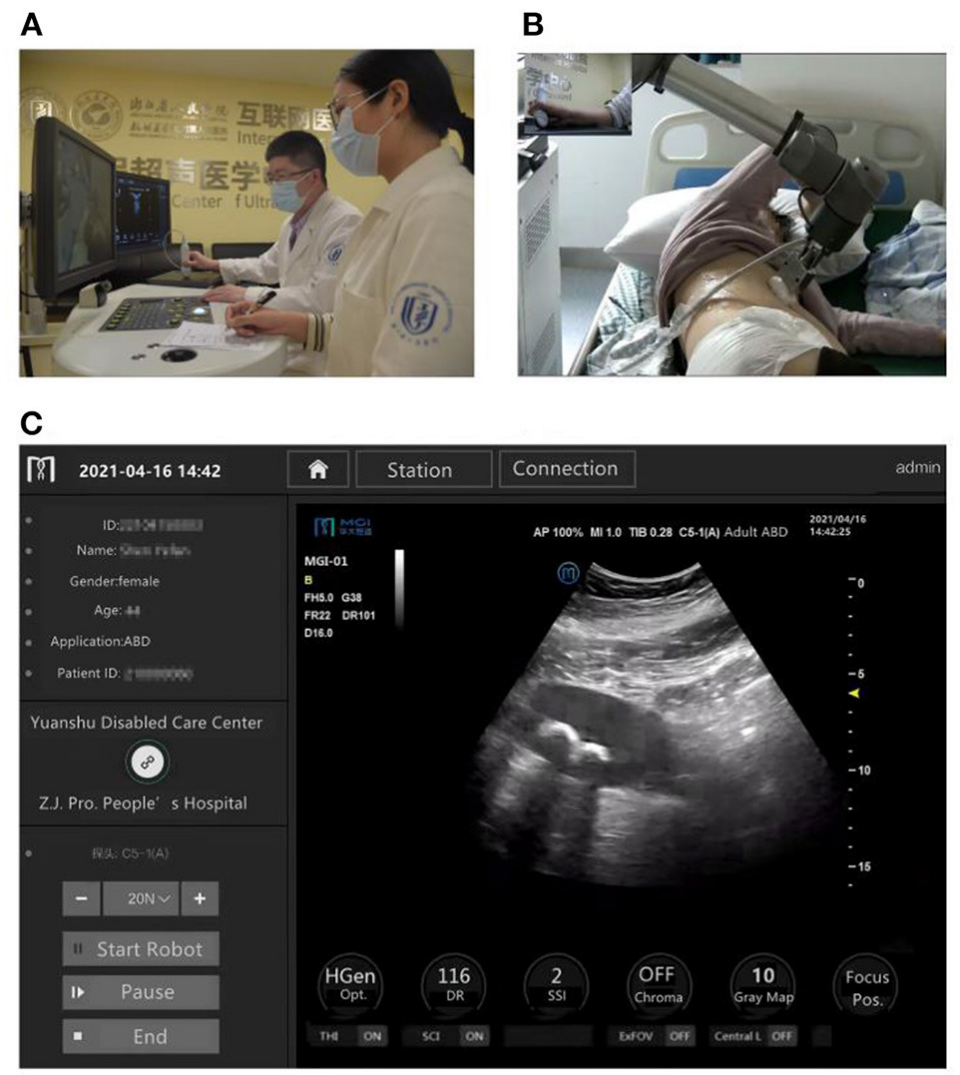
The 5G-based robot-assisted remote ultrasound used for patients in a rural care center for persons with disabilities in China. (A) The doctor-side operational scenario in Hangzhou. (B) The patient-side operational scenario in Deqing, 35.9 kilometers away from Hangzhou. (C) Ultrasound images captured from the patient-side subsystem were sent to the doctor-side subsystem in real time.
